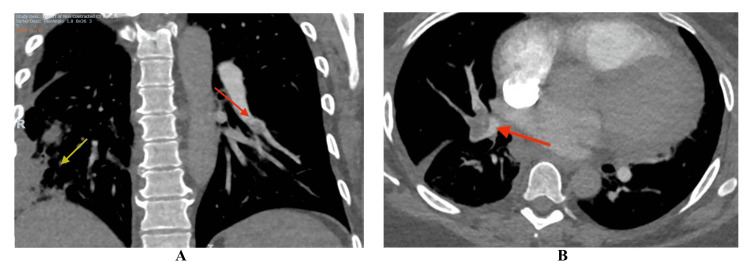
CTPA (pulmonary arterial phase, 10 seconds post-contrast injection) showing filling defects (red arrow) in the right and left interlobar arteries, along with right lung infarcts (yellow arrow) (A) CTPA (coronal section) showing focal areas of heterogeneous, wedge-shaped consolidation in the right lower lobe (yellow arrow) and filling defects in the left segmental pulmonary arteries (red arrow). (B) CTPA (transverse section) showing filling defects in the right segmental pulmonary arteries (red arrow). CTPA, CT of the pulmonary arteries
